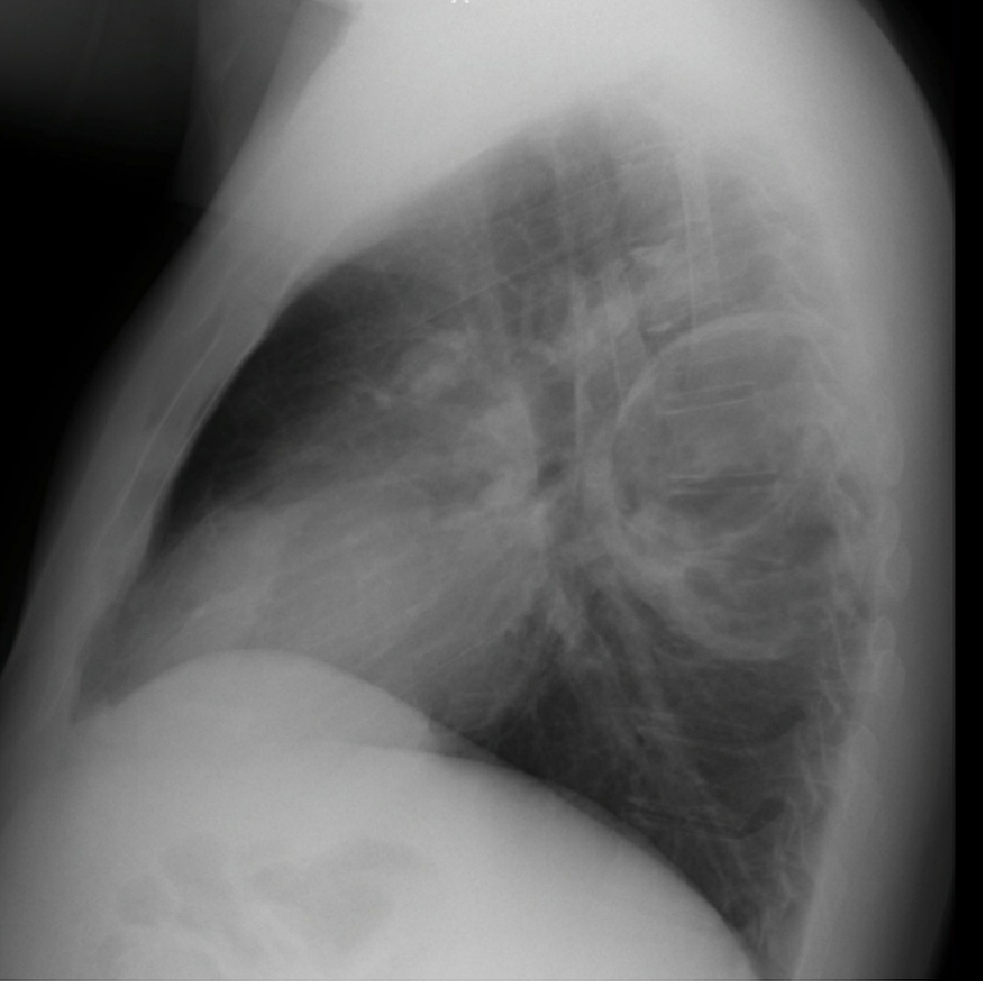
Lateral chest X-ray. A lateral chest X-ray confirmed a round lesion with defined borders on the left lower lung lobe.
